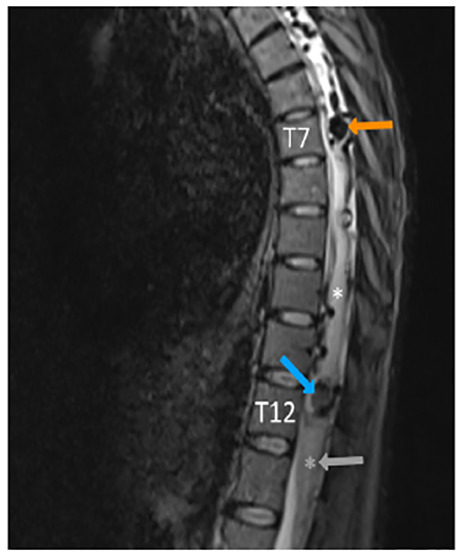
Sagittal magnetic resonance imaging T2-weighted image of the thoracic and lumbar spine. The orange arrow points to a flow void suggestive of an arteriovenous malformation with dilated varix at seventh thoracic (T7). There is a similar dilated varix at T11 to the twelfth thoracic (T12), and the blue arrow shows an area of darker gray suggesting hemosiderin from subacute or chronic hemorrhage. The spinal cord in this image is hyperintense due to venous congestion and edema, possibly worsened by ischemia. For comparison of relative signal intensity, the gray asterisk and arrow at the conus show the same signal level as the cord at the tenth thoracic level surrounding the white asterisk.
